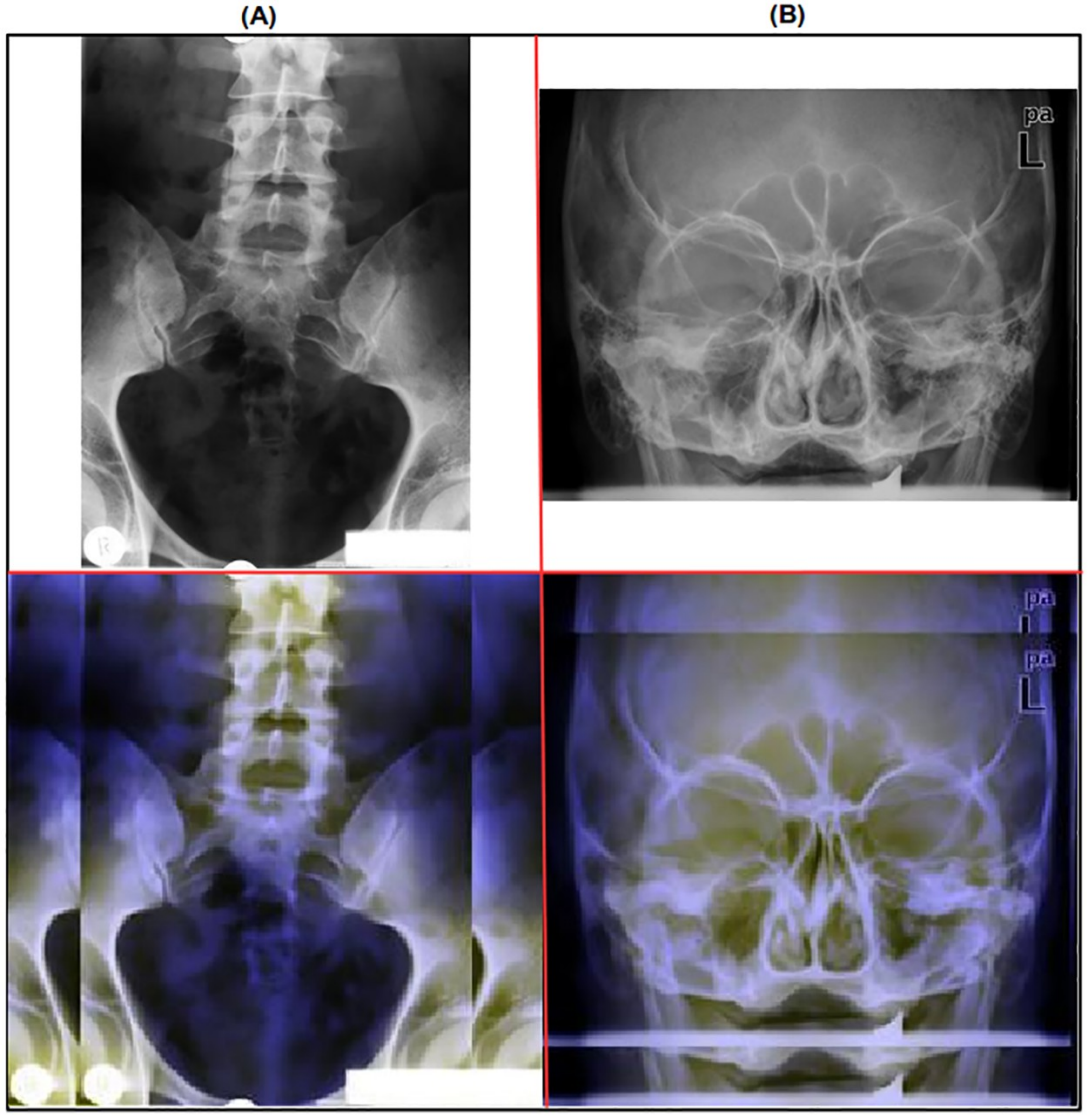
Output image after successively applying the image padding and image layering enhancement techniques. The radiographs were randomly chosen from the ImageCLEF 2009 Medical Annotation Task Training Set. Modified from [21] under a CC BY license, with permission from [RWTH Aachen], original copyright [2009].
